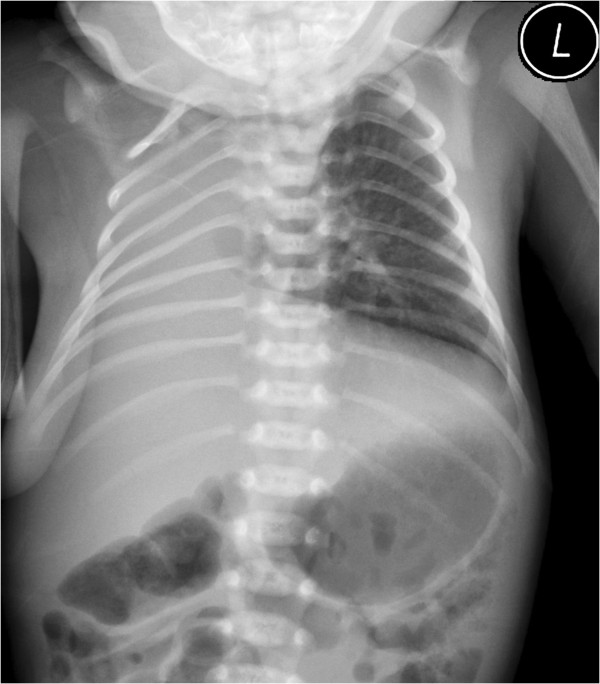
Digital radiographs of the chest showing an opaque, right hemithorax with mediastional shift to the left in a neonate that was diagnosed with right lung agenesis at 28 gestational weeks.
